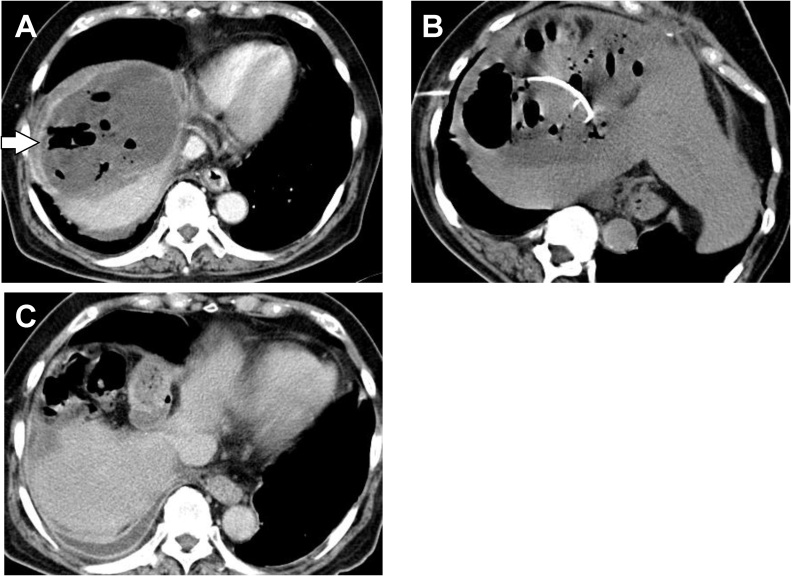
Computed tomography (CT) showed emphysematous subphrenic abscess on postoperative day POD 15 (A) and CT-guided percutaneous abscess drainage was performed on POD 16 (B). Subphrenic abscess disappeared on CT before the patient was discharged on POD 95 (C).
